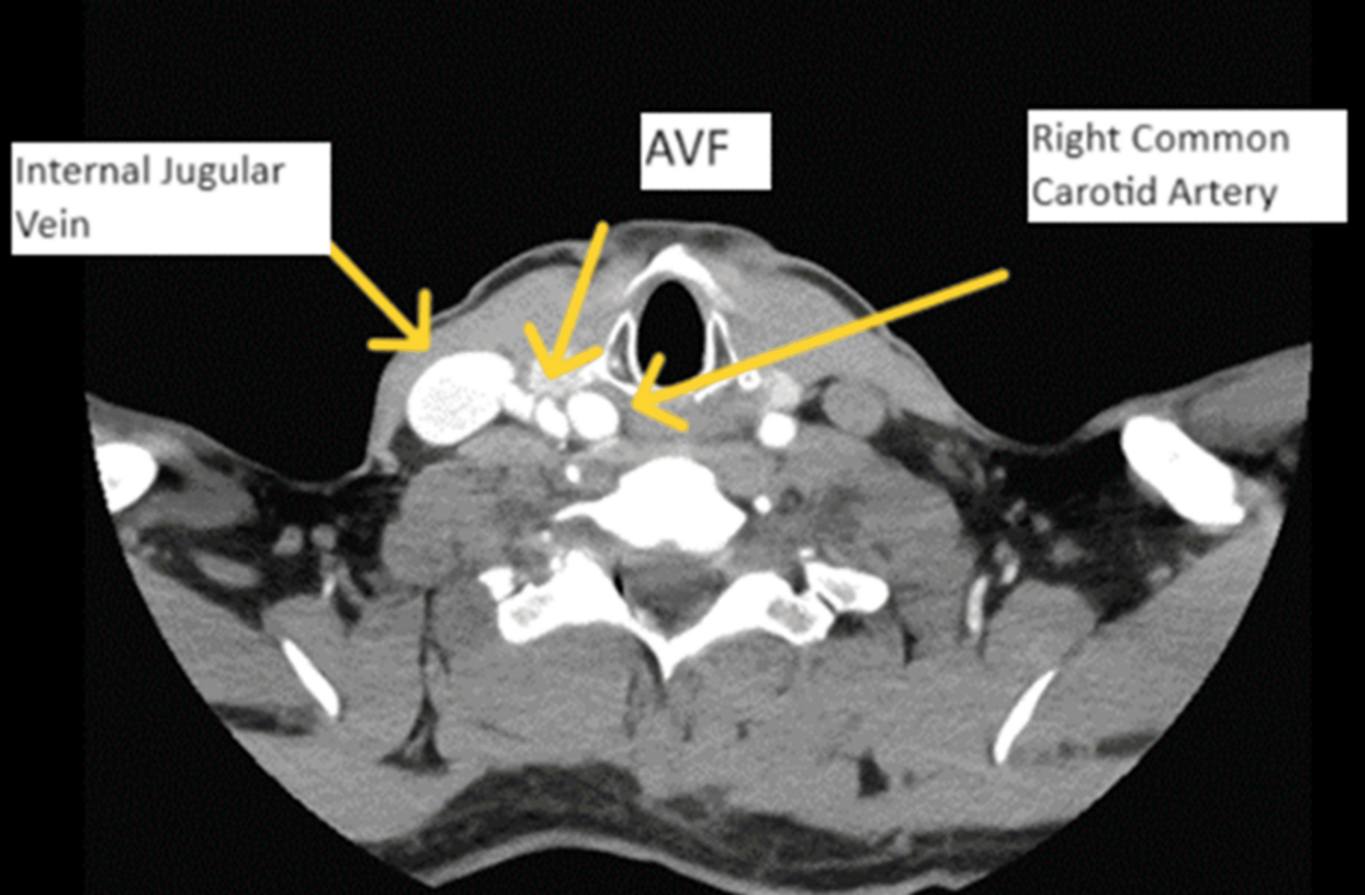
Computed tomography angiography head/neck with contrast depicting the carotid-jugular arteriovenous fistula AVF: arteriovenous fistula
